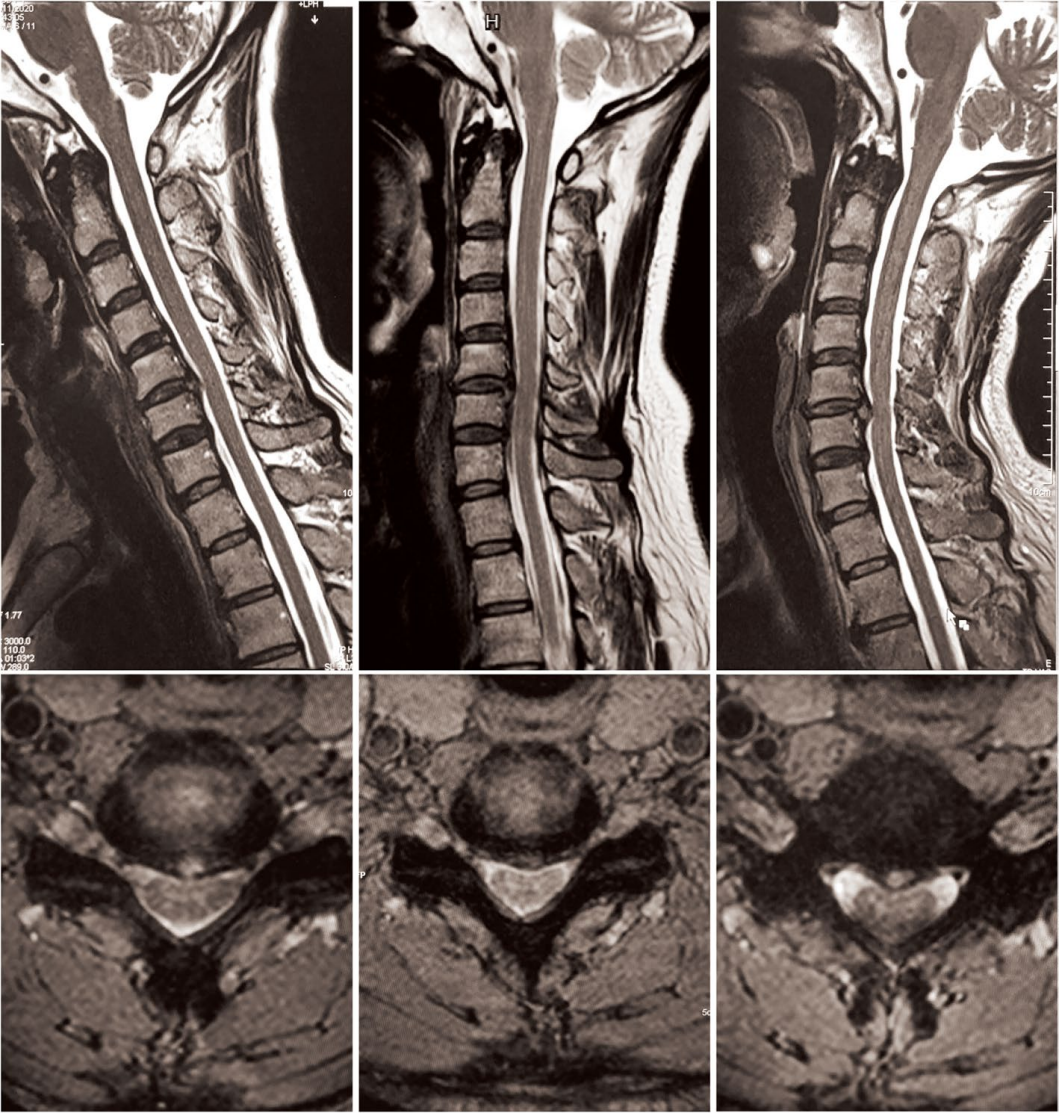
Dynamic MR images of a patient with improved DSSEPs at extension and deteriorated DSSEPs at flexion. Panels from left to right show cervical flexion, neutral and extension positions. Upon neutral positioning, this patient had a straight cervical alignment and a single protruding C5/6 segment. The Mühle stenosis grade of this patient was Grade 1. Upon flexion, the spinal cord was longitudinally stretched and draped backward. The cerebral fluid in front of the spinal cord was narrower in the axial image. Upon extension, although the diameter of his cervical canal did not change significantly, the spinal cord was longitudinally relaxed and draped backward and, therefore, ameliorated anterior compression to some extent. The cerebral fluid in front of the spinal cord was wider in the axial image captured in the extension position
